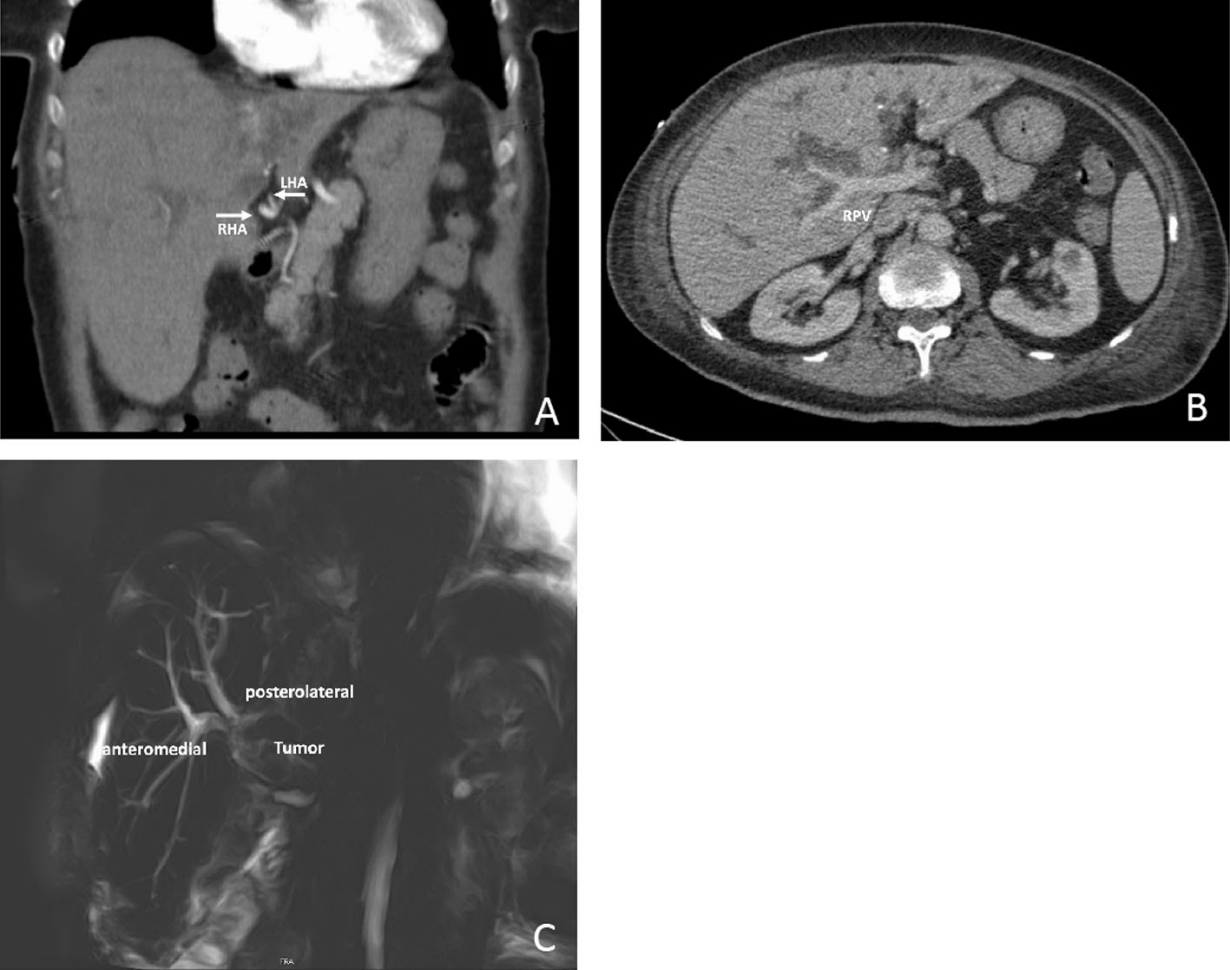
Preoperative computed tomography (CT) scan indicating no tumor infiltration of the a right hepatic artery (RHA) or b right portal vein (RPV). Magnetic resonance cholangiopancreatography (MRCP) suggests tumor-free anteromedial and posterolateral hepatic ducts. *LHA* left hepatic artery
